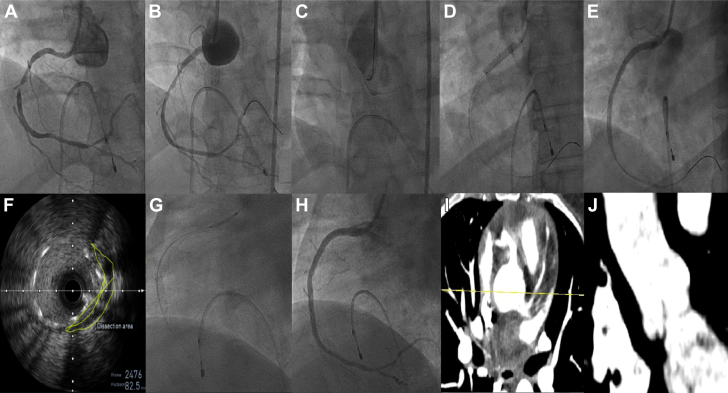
Angiographic, Ultrasonographic, and CT Images From Patient 1 Demonstrating Progression and Management of IACD (A) Angiogram showing a tight lesion in the mid-RCA. (B) Type III IACD postballooning. (C) Disengaged Judkins right catheter postevent. (D) Stenting of mid to proximal RCA after successful rewiring. (E) Persistent contrast extravasation poststenting. (F) IVUS of RCA ostium showing incomplete coverage. (G) Additional stenting extended into the aortic sinus. (H) Final angiogram showing sealed-off dissection with good distal flow. (I and J) CT aortogram (transverse and coronal) confirming sealed-off aortic dissection without further propagation. CT = computed tomography; IACD = iatrogenic aortocoronary dissection; IVUS = intravascular ultrasound; RCA = right coronary artery.
